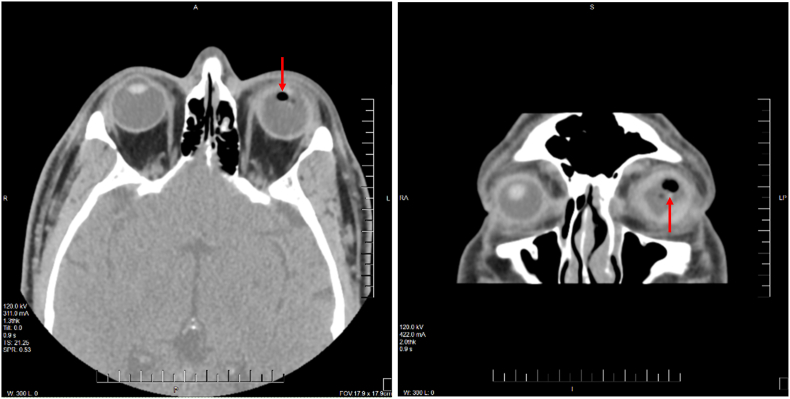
Computed tomography orbits without intravenous contrast. A. Axial cut soft tissue window demonstrating intralenticular “air” (arrow) in the left eye. B. Coronal cut soft tissue window demonstrating intralenticular “air” (arrow) in the left eye. The term “air” is noted in quotations because the trapped gas within the IOFB limits the plastic component from being seen on CT.
